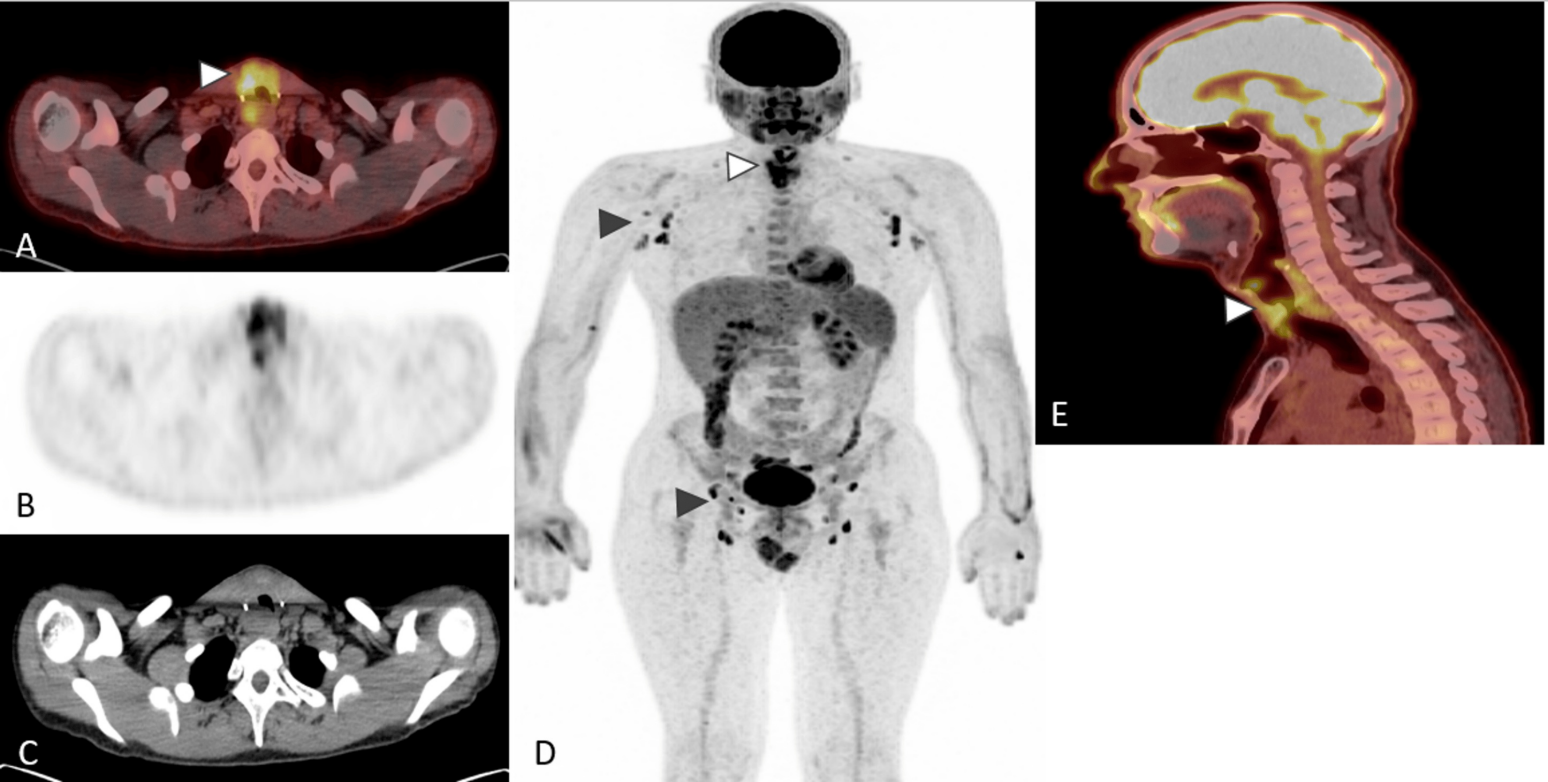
PET/CT images Axial fused PET/CT images (A, B, C) demonstrating, by the white arrowhead, a hypermetabolic right posterior subglottic residual mass invading the trachea (SUVmax = 8.6). (D) Maximum intensity projection (MIP) image demonstrates a hypermetabolic right posterior subglottic residual mass invading the trachea (maximum standardized uptake value (SUVmax) = 8.6) demonstrated by the white arrowhead, hypermetabolic metastatic bilateral cervical lymph nodes (SUVmax = 4.6), and multiple hypermetabolic bilateral axillary, external iliac, and bilateral inguinal lymph nodes, demonstrated by the two black arrowheads (SUVmax = 7.1). Sagittal fused PET/CT image (E) shows the subglottic lesion invading the adjacent trachea, demonstrated by the white arrowhead.
